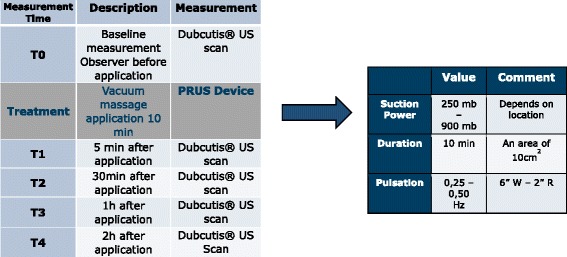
Assessment protocol with inclusion of the treatment protocol of the vacuum massage. *US* ultrasound, *mb* millibar, *Hz* Hertz, *W* working time, *R* resting time, *”* seconds
